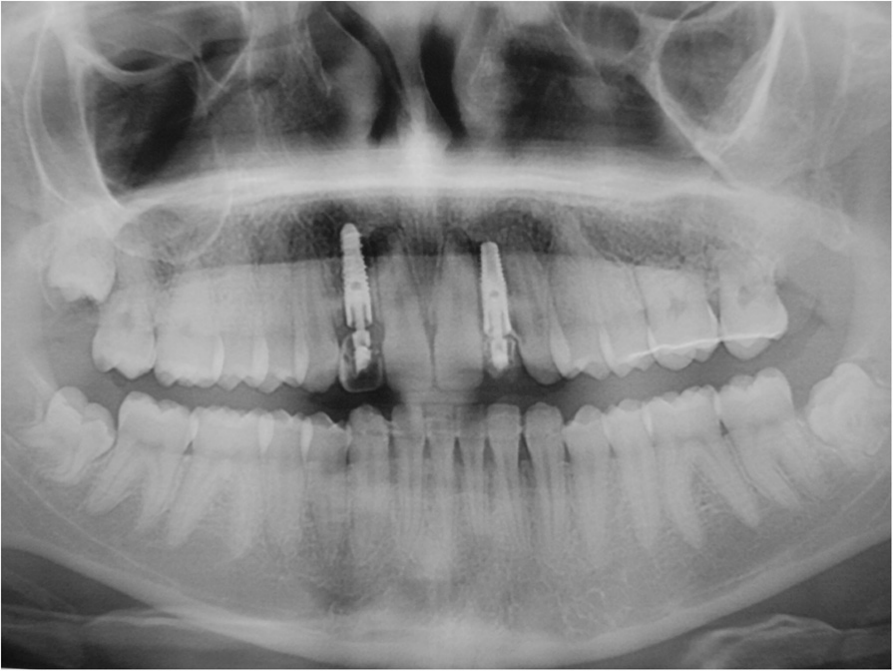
Orthopantomography examination at 24 months.
